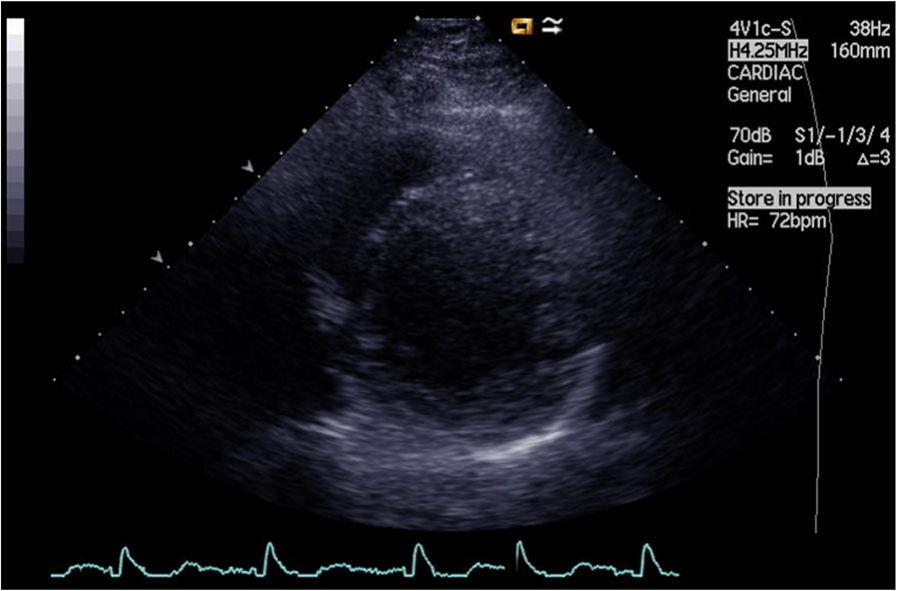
Transthoracic echocardiogram, end-diastole, parasternal short axis view showing hypertrabeculation of the inferolateral left ventricular wall
